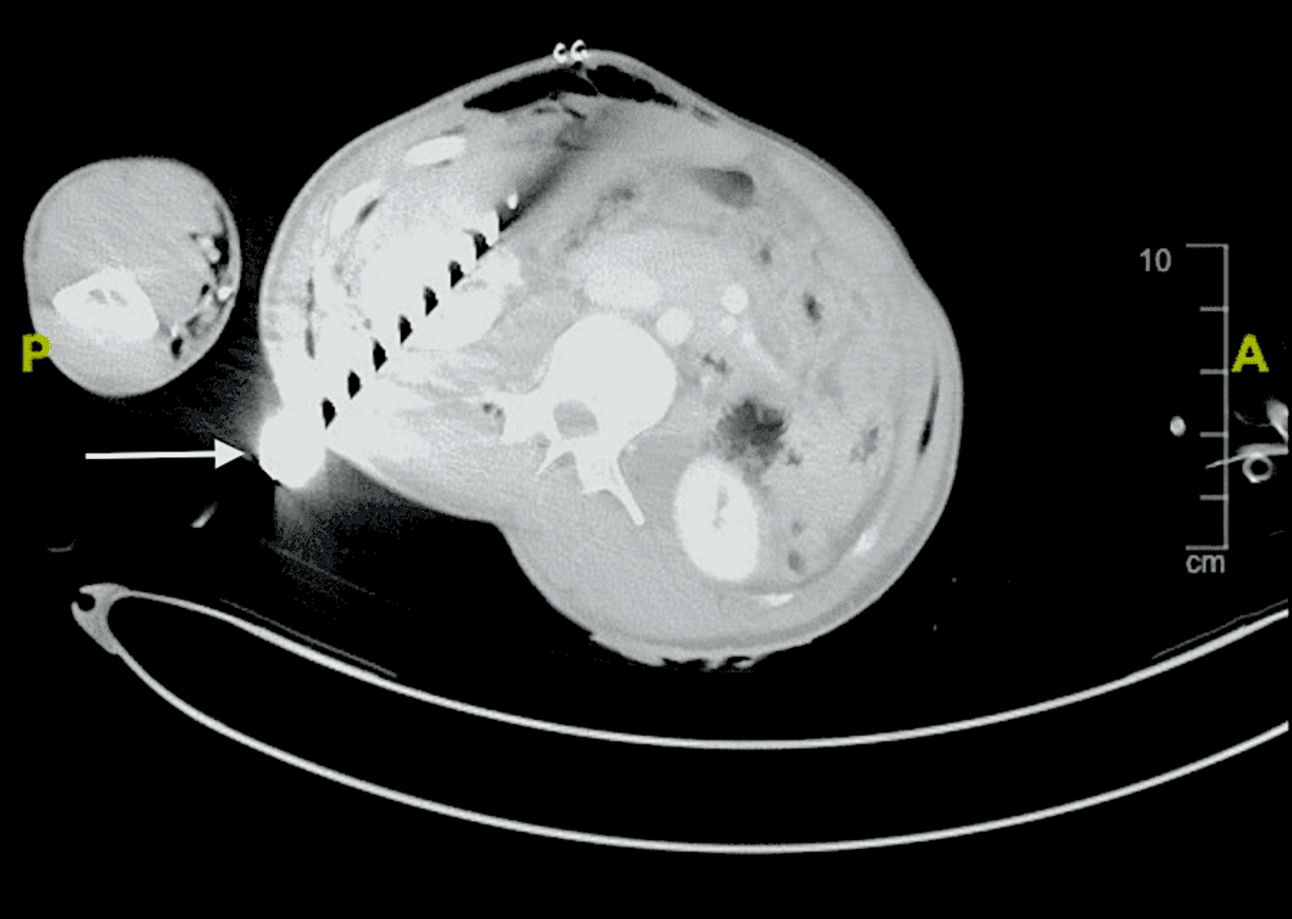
CT scan of the abdomen showing impaled sharp knife (white arrow) through the lower pole of the right kidney sparing the right renal pedicle CT: computed tomography
